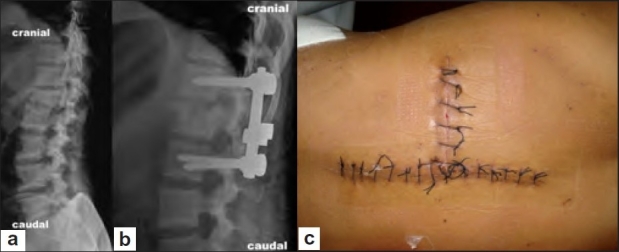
(a) Preoperative X-ray lateral view showing unstable burst fracture (type B) of L1 vertebra. (b) Immediate postoperative X-ray lateral view of the same patient after pedicle screw fixation, anterior decompression, and bone grafting via single-stage extrapleural retroperitoneal approach by “T” incision. (c) Clinical photograph of the “T” incision after 2 week of surgery
